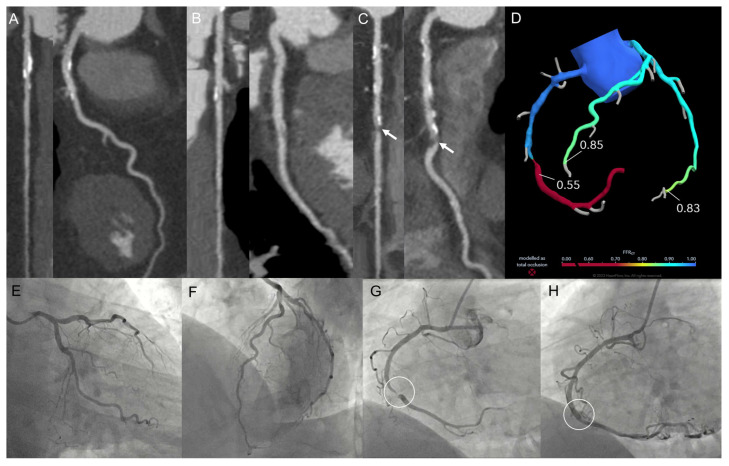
A 60-year-old man, former smoker, with dyslipidemia and a family history of CAD, presented with an onset of effort chest pain. CCT demonstrated mild stenoses at left anterior descending (A) and left circumflex arteries (B) and a severe stenosis at mid-right coronary artery (arrows; (C)). Pathological FFR-CT assessment at mid-right coronary artery; normal FFR-CT values at distal left circumflex and left anterior descending arteries (D). ICA confirmed non-obstructive disease of the left circumflex and left anterior descending arteries (E,F) and severe stenosis of the mid-right coronary artery (white circle; (G)). The patient was treated with percutaneous coronary intervention and drug-eluting stent implantation (white circle; (H)).
